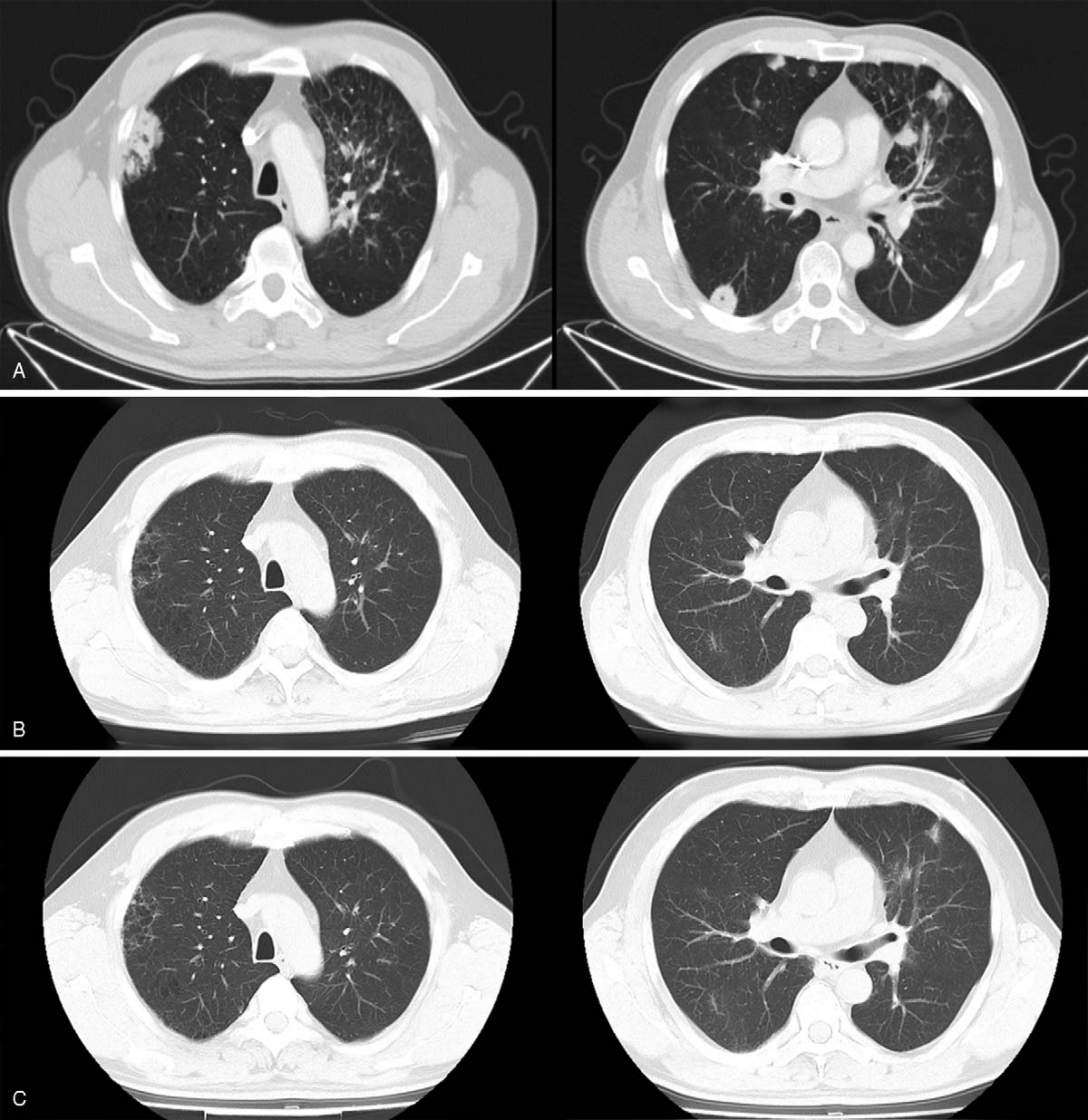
Chest CT imaging results for the case 1 during treatment with dacomitinib. (A) Chest CT scans revealed a high-density shadow in the left upper lobe, accompanied by multiple nodules in both lungs at diagnose (December 26, 2019). (B) Two months after treatment, the mass in the upper lobe of the left lung was significantly reduced in size, and the bilateral pulmonary nodules almost disappeared. (C) At the time of disease progresses (November 29, 2020), the volume and number of multiple pulmonary nodules in both lungs were increased, the mass in the upper lobe of the left lung remained stable. CT = computed tomography.
